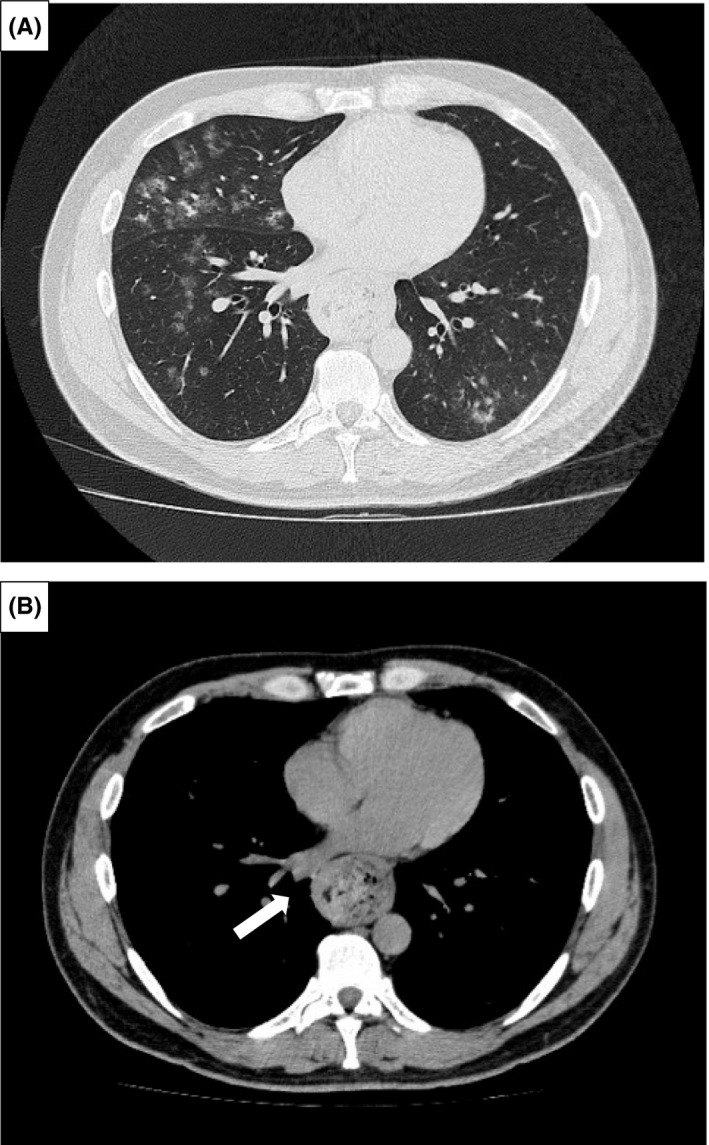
Axial CT scans of the chest (A: lung window; B: mediastinal window) showing small nodular shadow in both lungs and dilation of a food‐filled esophagus
